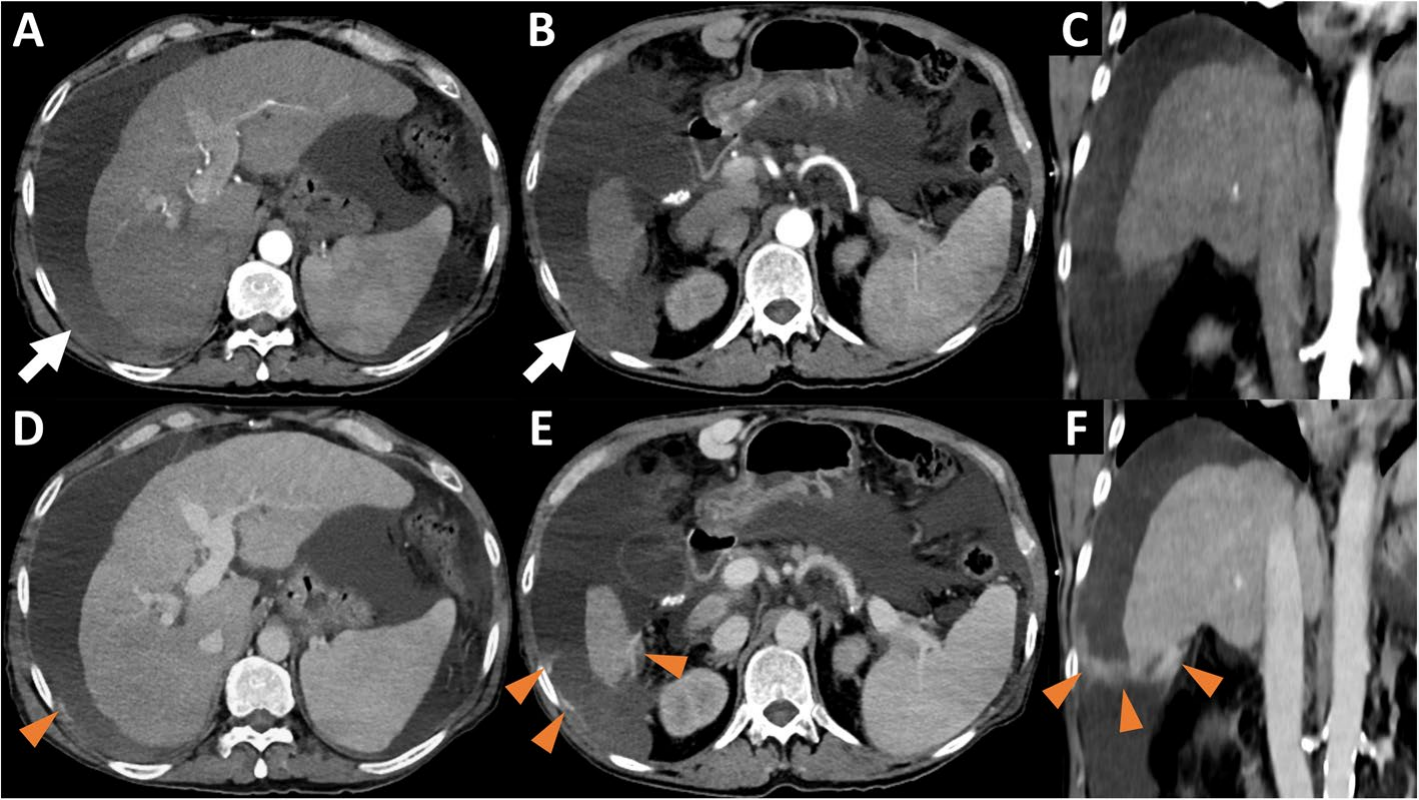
Pre-interventionnal Computed Tomography. Arterial phase in axial (A, B) and coronal (C) sections and portal phase in axial (D, E) and coronal (F) sections showing liver cirrhosis with a large intraperitoneal effusion, with perihepatic hyperdensity (white arrows) testifying to hemoperitoneum, without active bleeding in the arterial phase but with extravasation of contrast agent in the portal phase (orange arrowheads)
